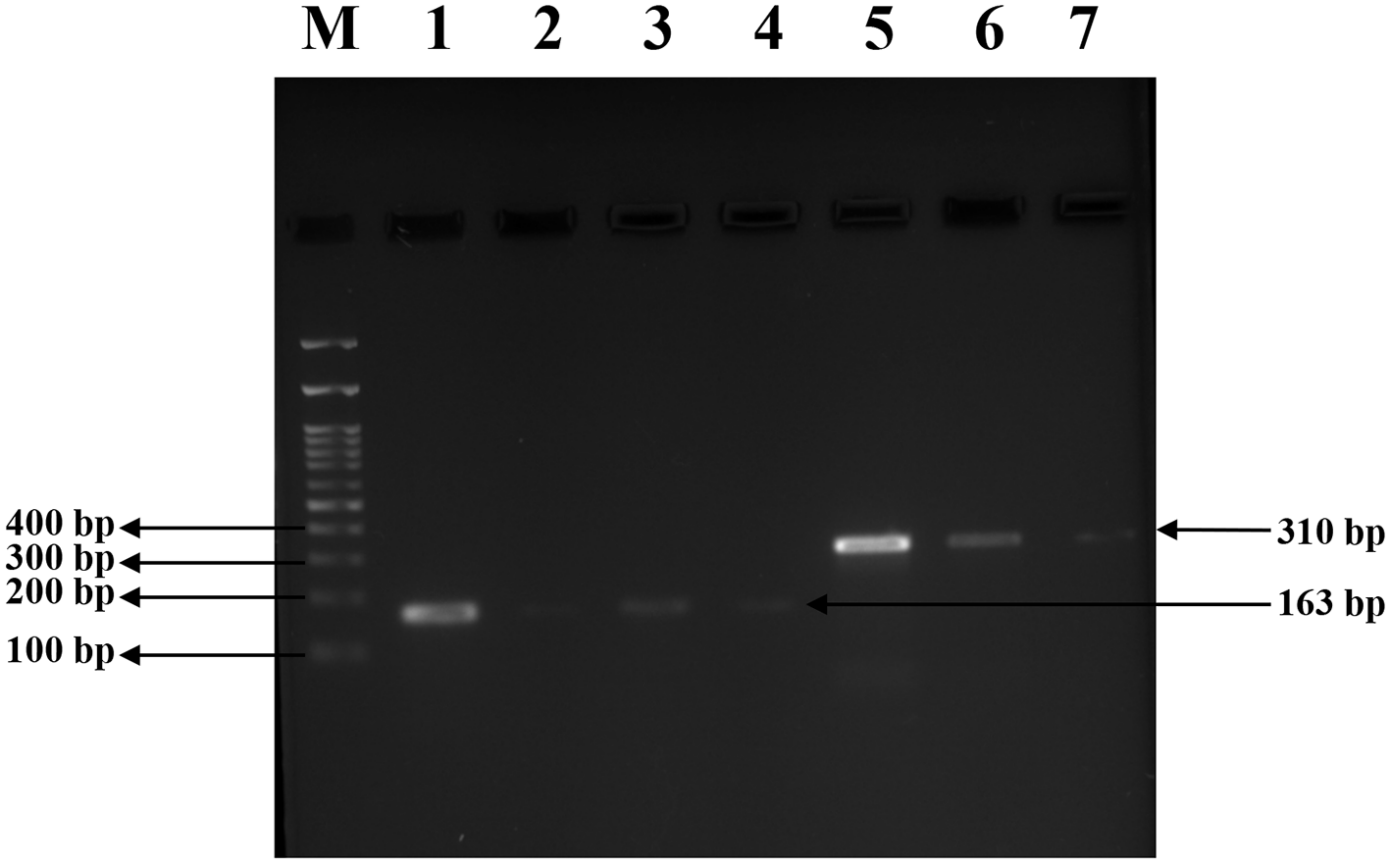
Agarose gel electrophoresis for visualizing the downregulated gene expression following BACs treatment. Gel image indicating PCR products of *mecA* (310 bp) and *agrA* (163 bp) amplified from treated *S. aureus* strains. Lane M: 100 bp DNA ladder; Lane 1: positive control for *agrA* (ATCC43300); Lanes 2, 3 and 4: *agrA* amplification from VITKV25 (CT), and MRSA (CT), and VITKV32 (ET), respectively; Lane 5: positive control for *mecA* (ATCC 43300); Lane 6,7: *mecA* amplification from VITKV39 (CT) and VITKV32 (ET), respectively. Reduced band intensity in treated samples indicates transcriptional suppression of target genes.
